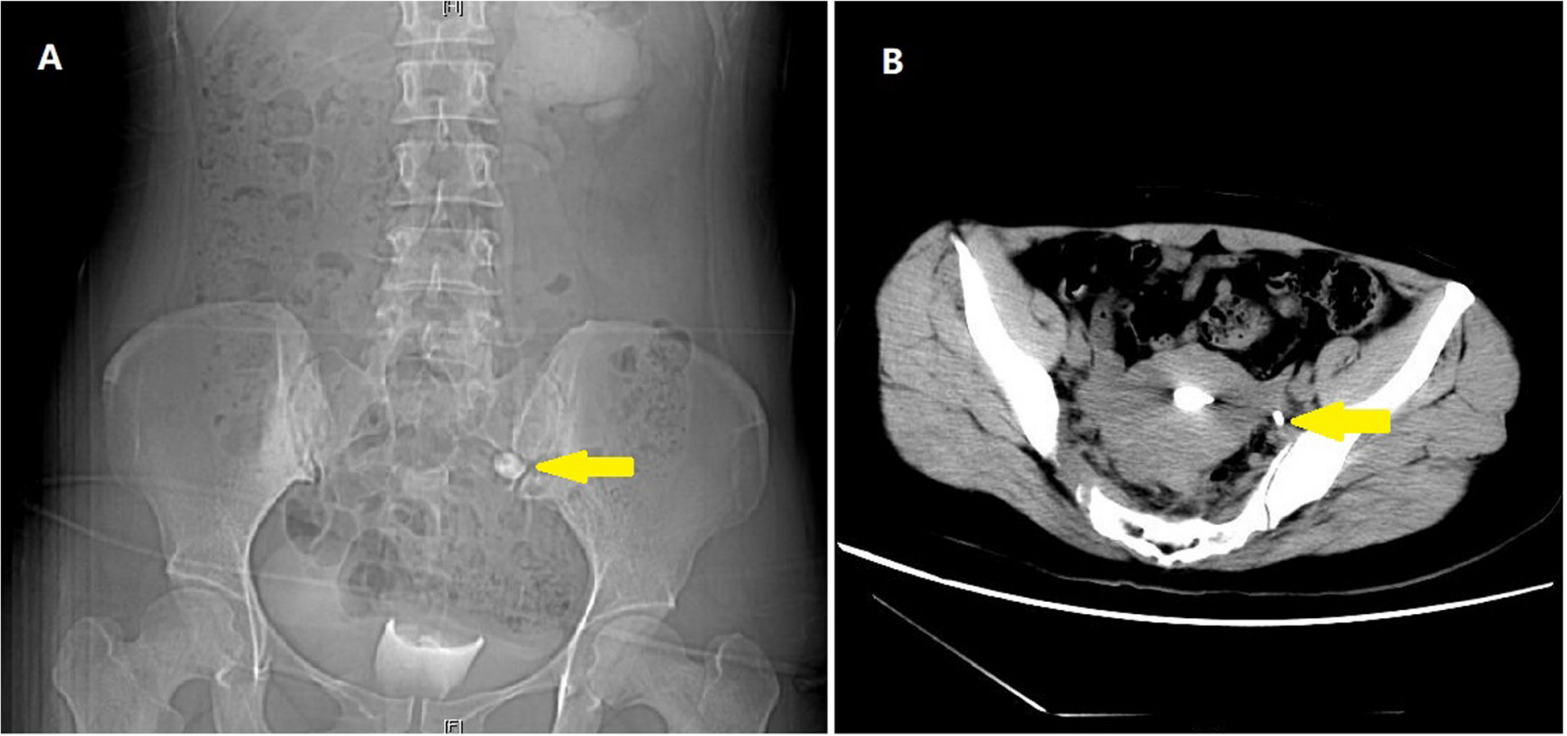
Retrograde CT ureterogram. a Contrast media reached left proximal ureter and left pelvis (Arrow: uretero-fallopian adhesion). b Contrast media was seen in uterus (Arrow: uretero-fallopian adhesion)
